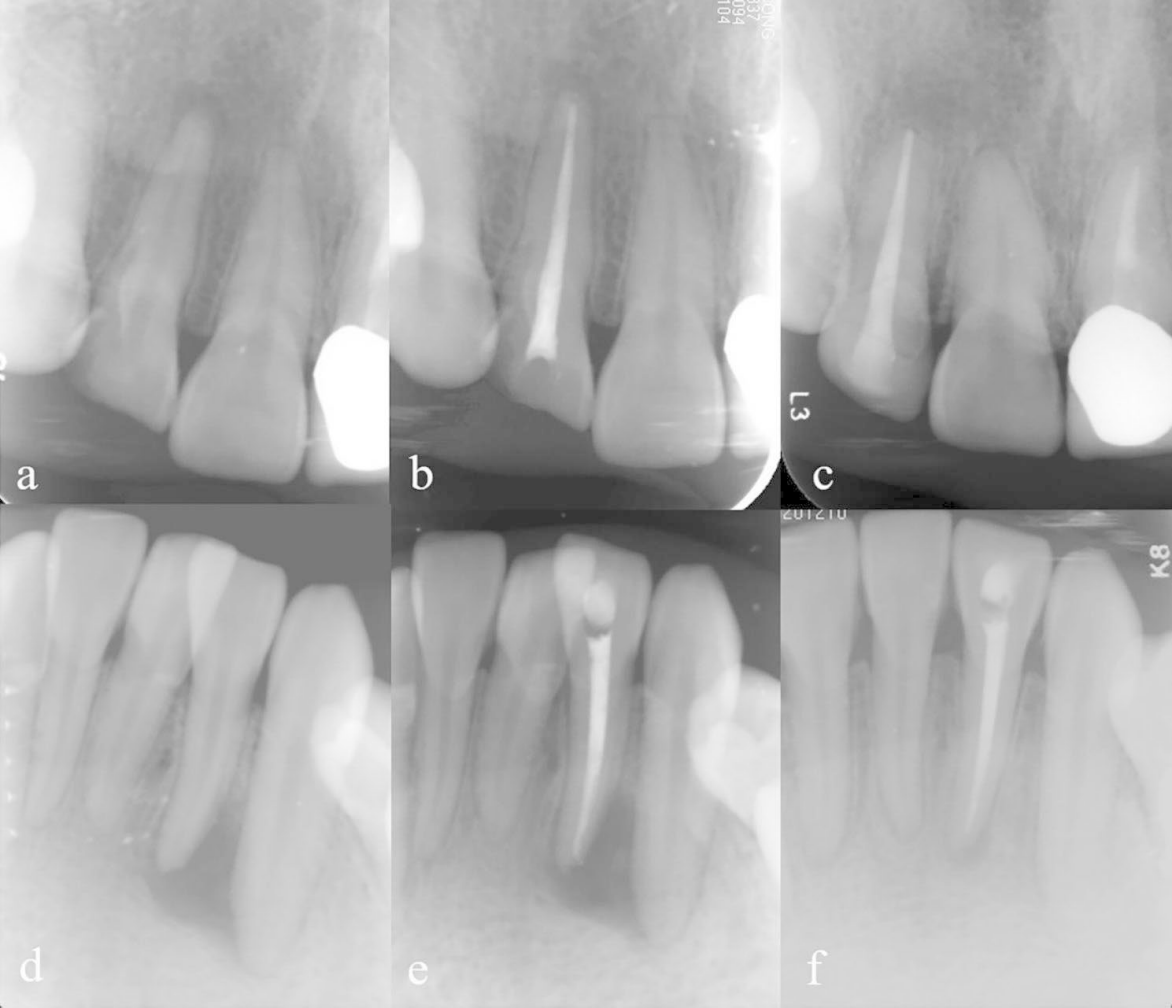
Representative successful cases of nonsurgical root canal treatment (NSRCT). (a-c) A 36-year-old female with a periapical lesion on right maxillary lateral incisor. (a) received root canal treatment and resin restoration (b). A radiograph of tooth 12 showed a flush root filling and no void was observed. There was no clinical signs or symptoms at 5 years recall after treatment. The postoperative PA images revealed resolved periapical lesions (c) and the case was determined as success. (d-f) The preoperative (d) and postoperative (e) PA images of tooth 32, which was diagnosed as apical periodontitis and underwent root canal treatment and received resin restoration. The periapical radiolucency reduced at the 5-year follow-up evaluation (f) and the case was determined as success
